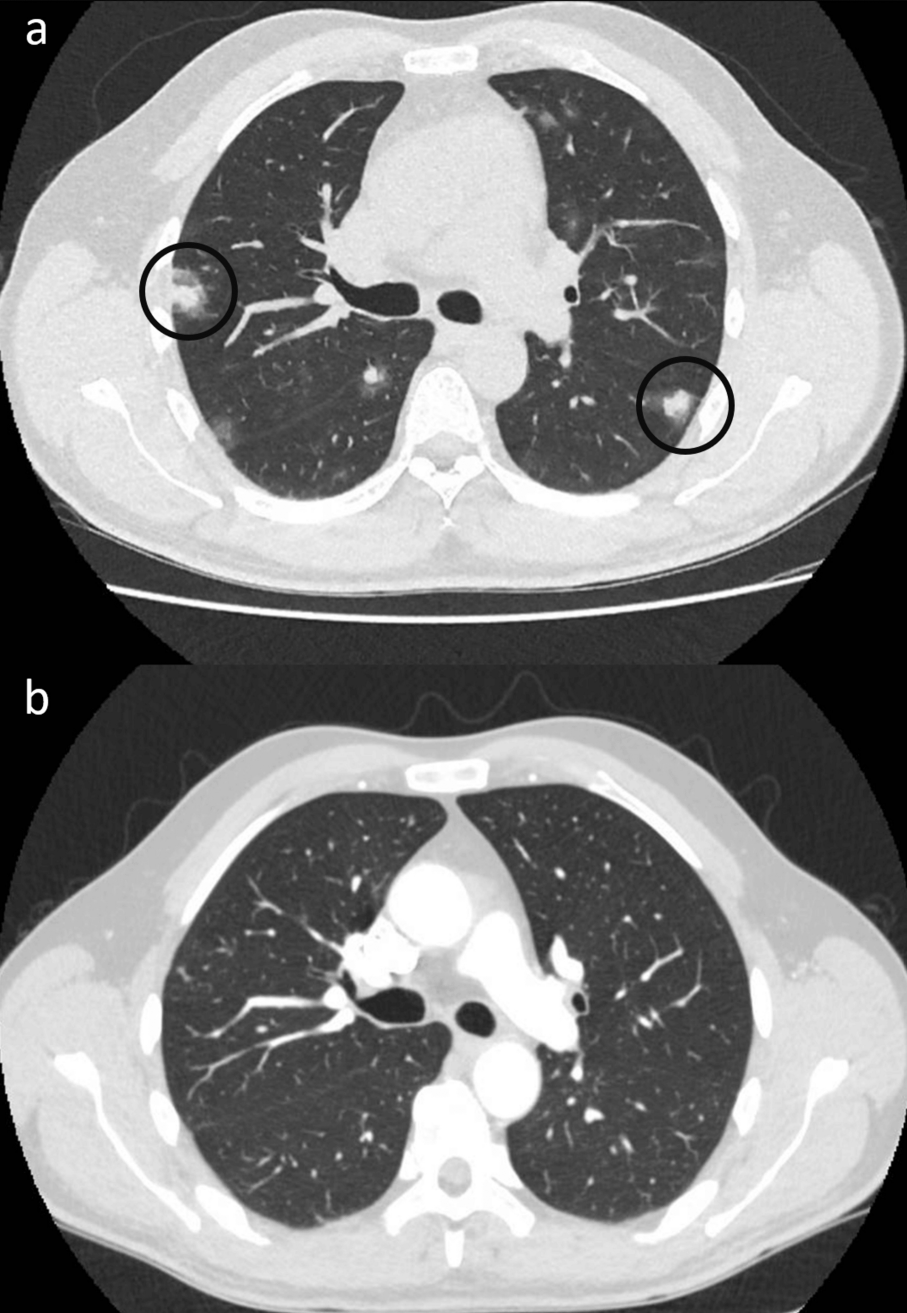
Computed tomography (CT) comparison of lung metastases (identified by circles) before systemic therapy (a) and on restaging imaging post-cycle six chemotherapy (b)
